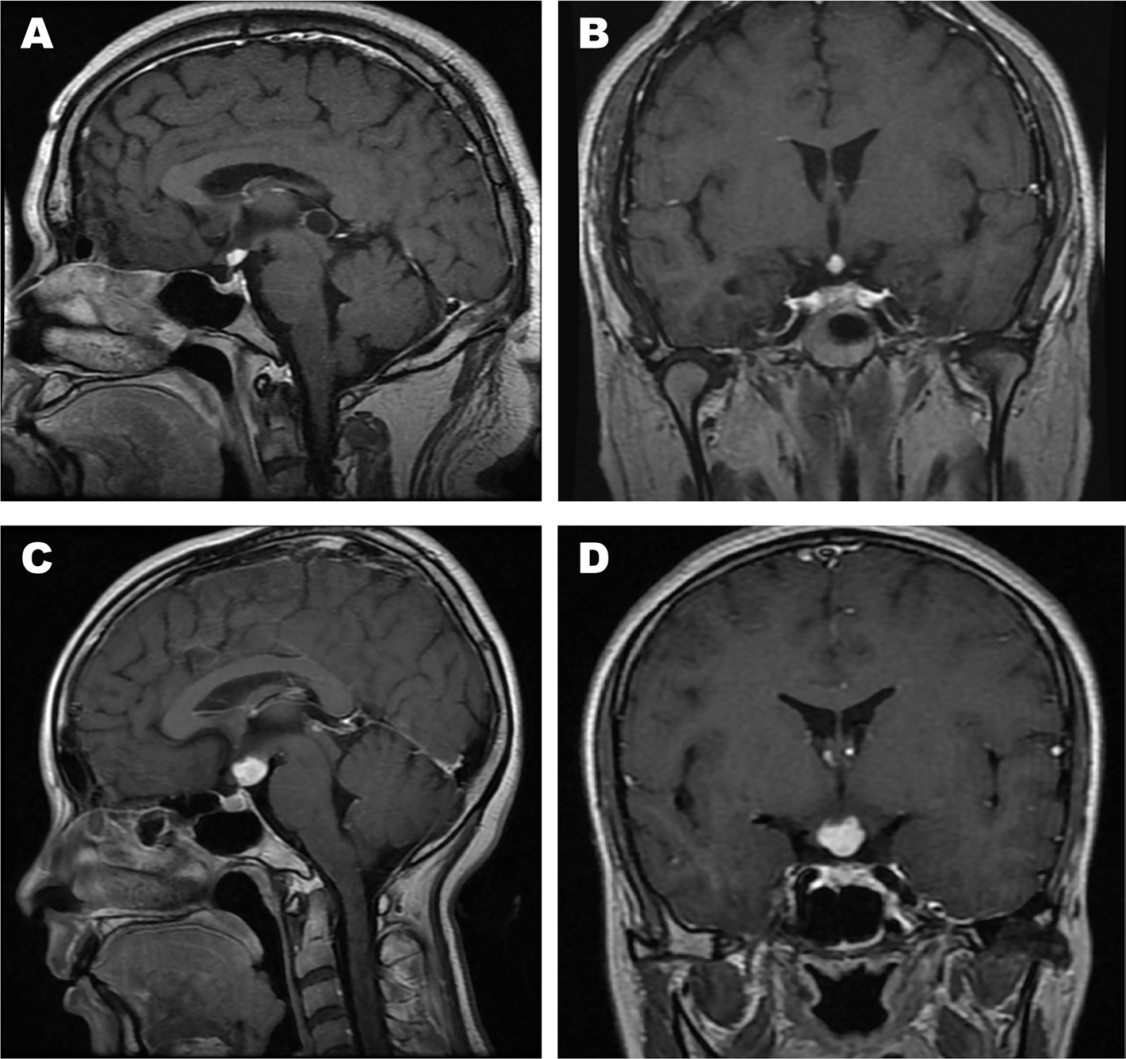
The T1-weighted postcontrast MR images of two typical cases. (A) Sagittal view of patient No. 7; (B) Coronal view of patient No. 7; (C) Sagittal view of patient No. 10; (D) Coronal view of patient No. 10.
